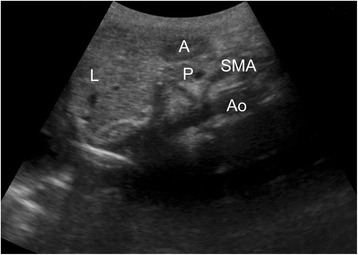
Sagittal sonographic image of the gastric antrum. A = antrum; P = pancreas; L = liver; SMA = superior mesenteric artery; Ao = aorta
